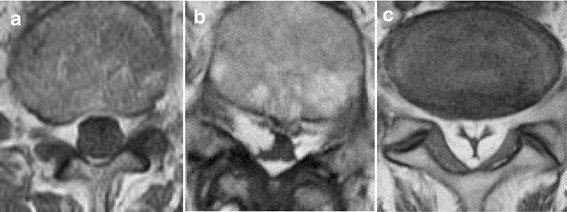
Classification of shape of dural sac on axial T1-weighted magnetic resonance imaging. a Circular type, b square stellate type, c “Y-sign”
